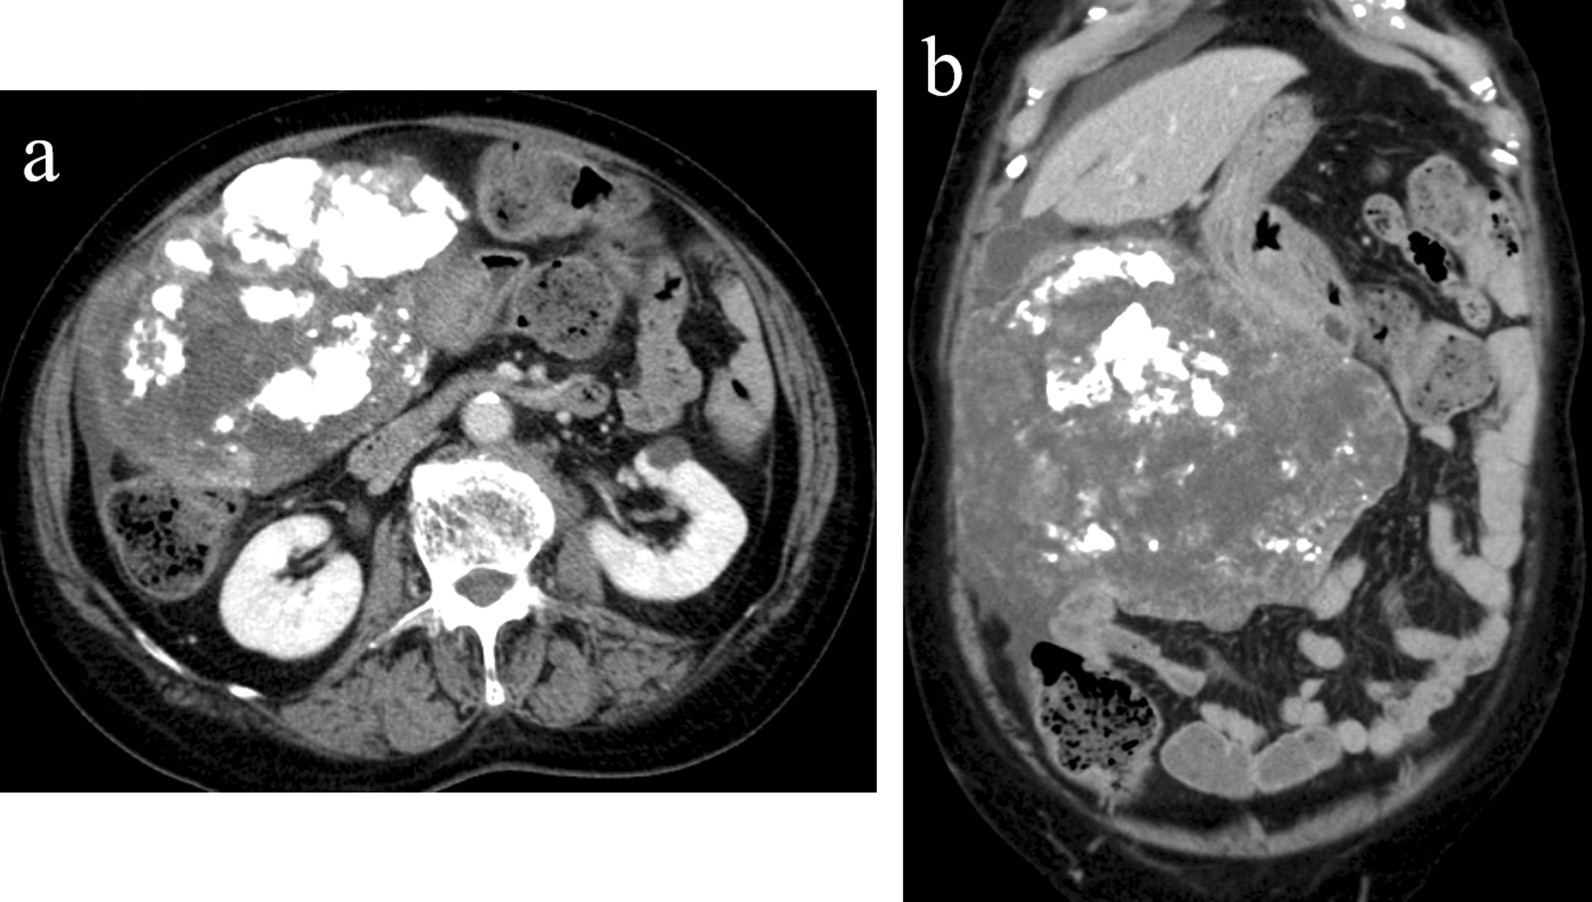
a, b Enhanced computed tomography reveals that the tumor had shrunk to approximately 18 cm and the stomach was twisted organoaxially
